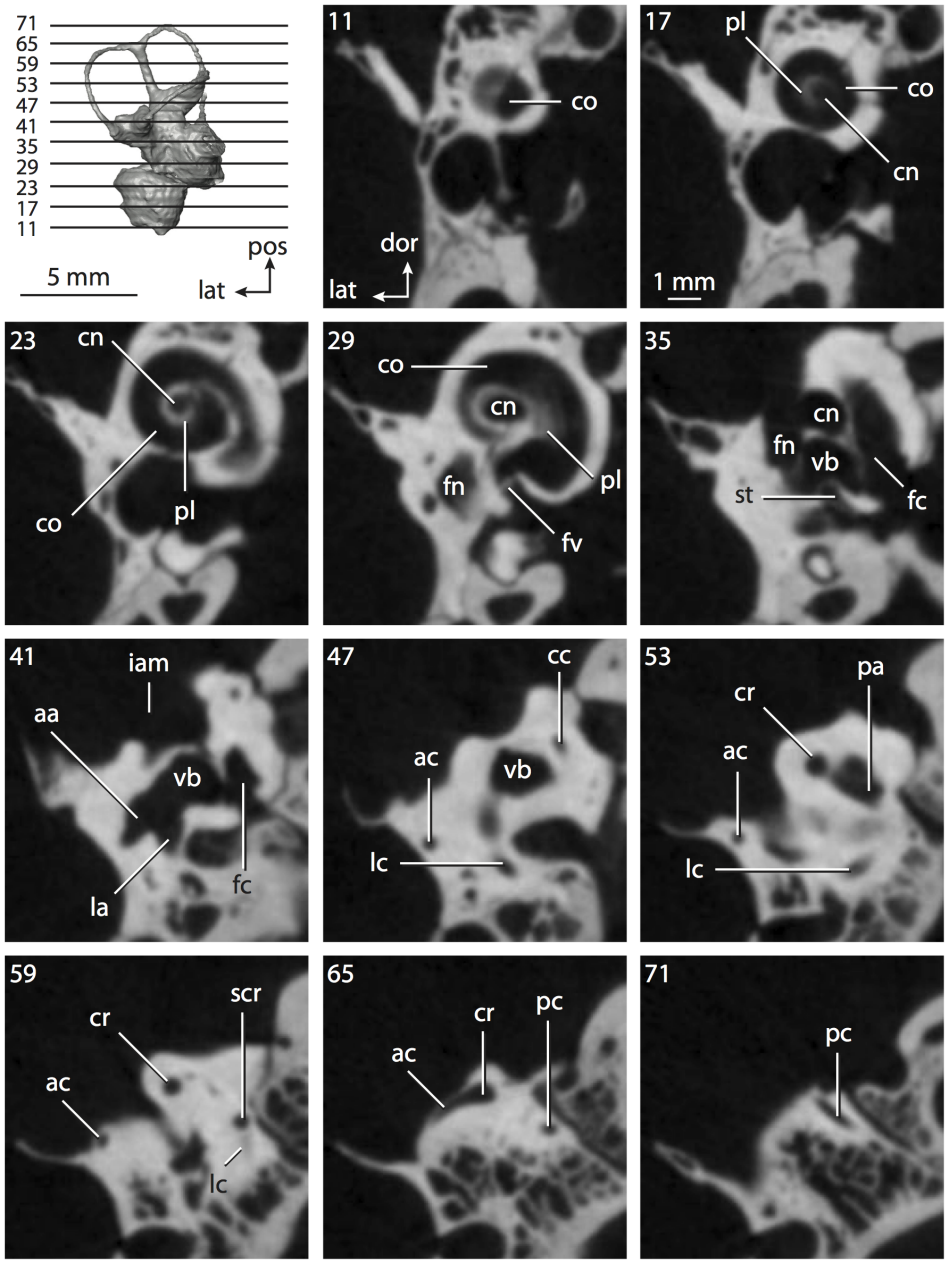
CT slices through ear region of*Canis familiaris*. Abbreviations listed at the end of the Materials and Methods section.
